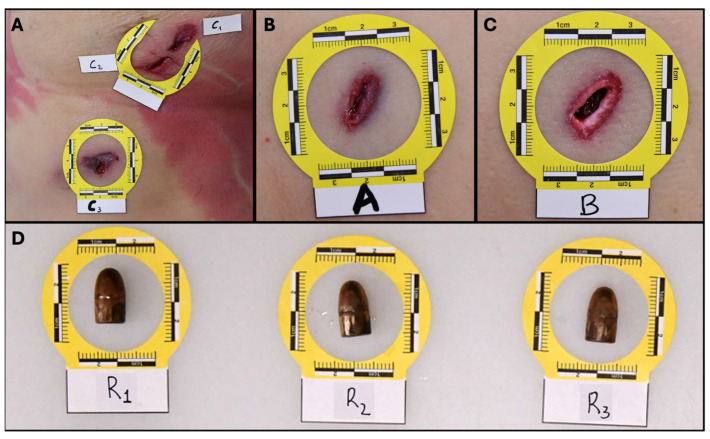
Thoracic gunshot wounds and retained bullets relative to Case 1. (A) Three distinct wounds from Bullet C are documented: Entrance Wound C1 (lower left breast), Exit Wound C2 (adjacent to C1), and Entrance Wound C3 (lower chest). The wounds show varied characteristics, with C1 and C3 presenting inwardly folded edges and reddish-brown discoloration, while C2 displays outwardly folded edges, indicating it as an exit wound; (B) Entrance Wound A from Bullet A located on the left back at the level of the first lumbar vertebra. The wound is oval-shaped, with finely hemorrhagic edges and surrounded by a reddish-brown area and an ecchymotic halo; (C) Entrance Wound B from Bullet B, positioned at the left back at the level of the seventh thoracic vertebra, shows a triangular shape with inwardly folded edges, surrounded by a reddish-brown area and an ecchymotic halo.; (D) Retained bullets (R1, R2, R3) corresponding to each shot fired. These retained bullets were confirmed through CT scans and autopsy findings, supporting the conclusion that all wounds, except C2, represent entrance wounds. The irregular wound shapes suggest instability and rotation of the bullets during flight, leading to side-on impacts.
